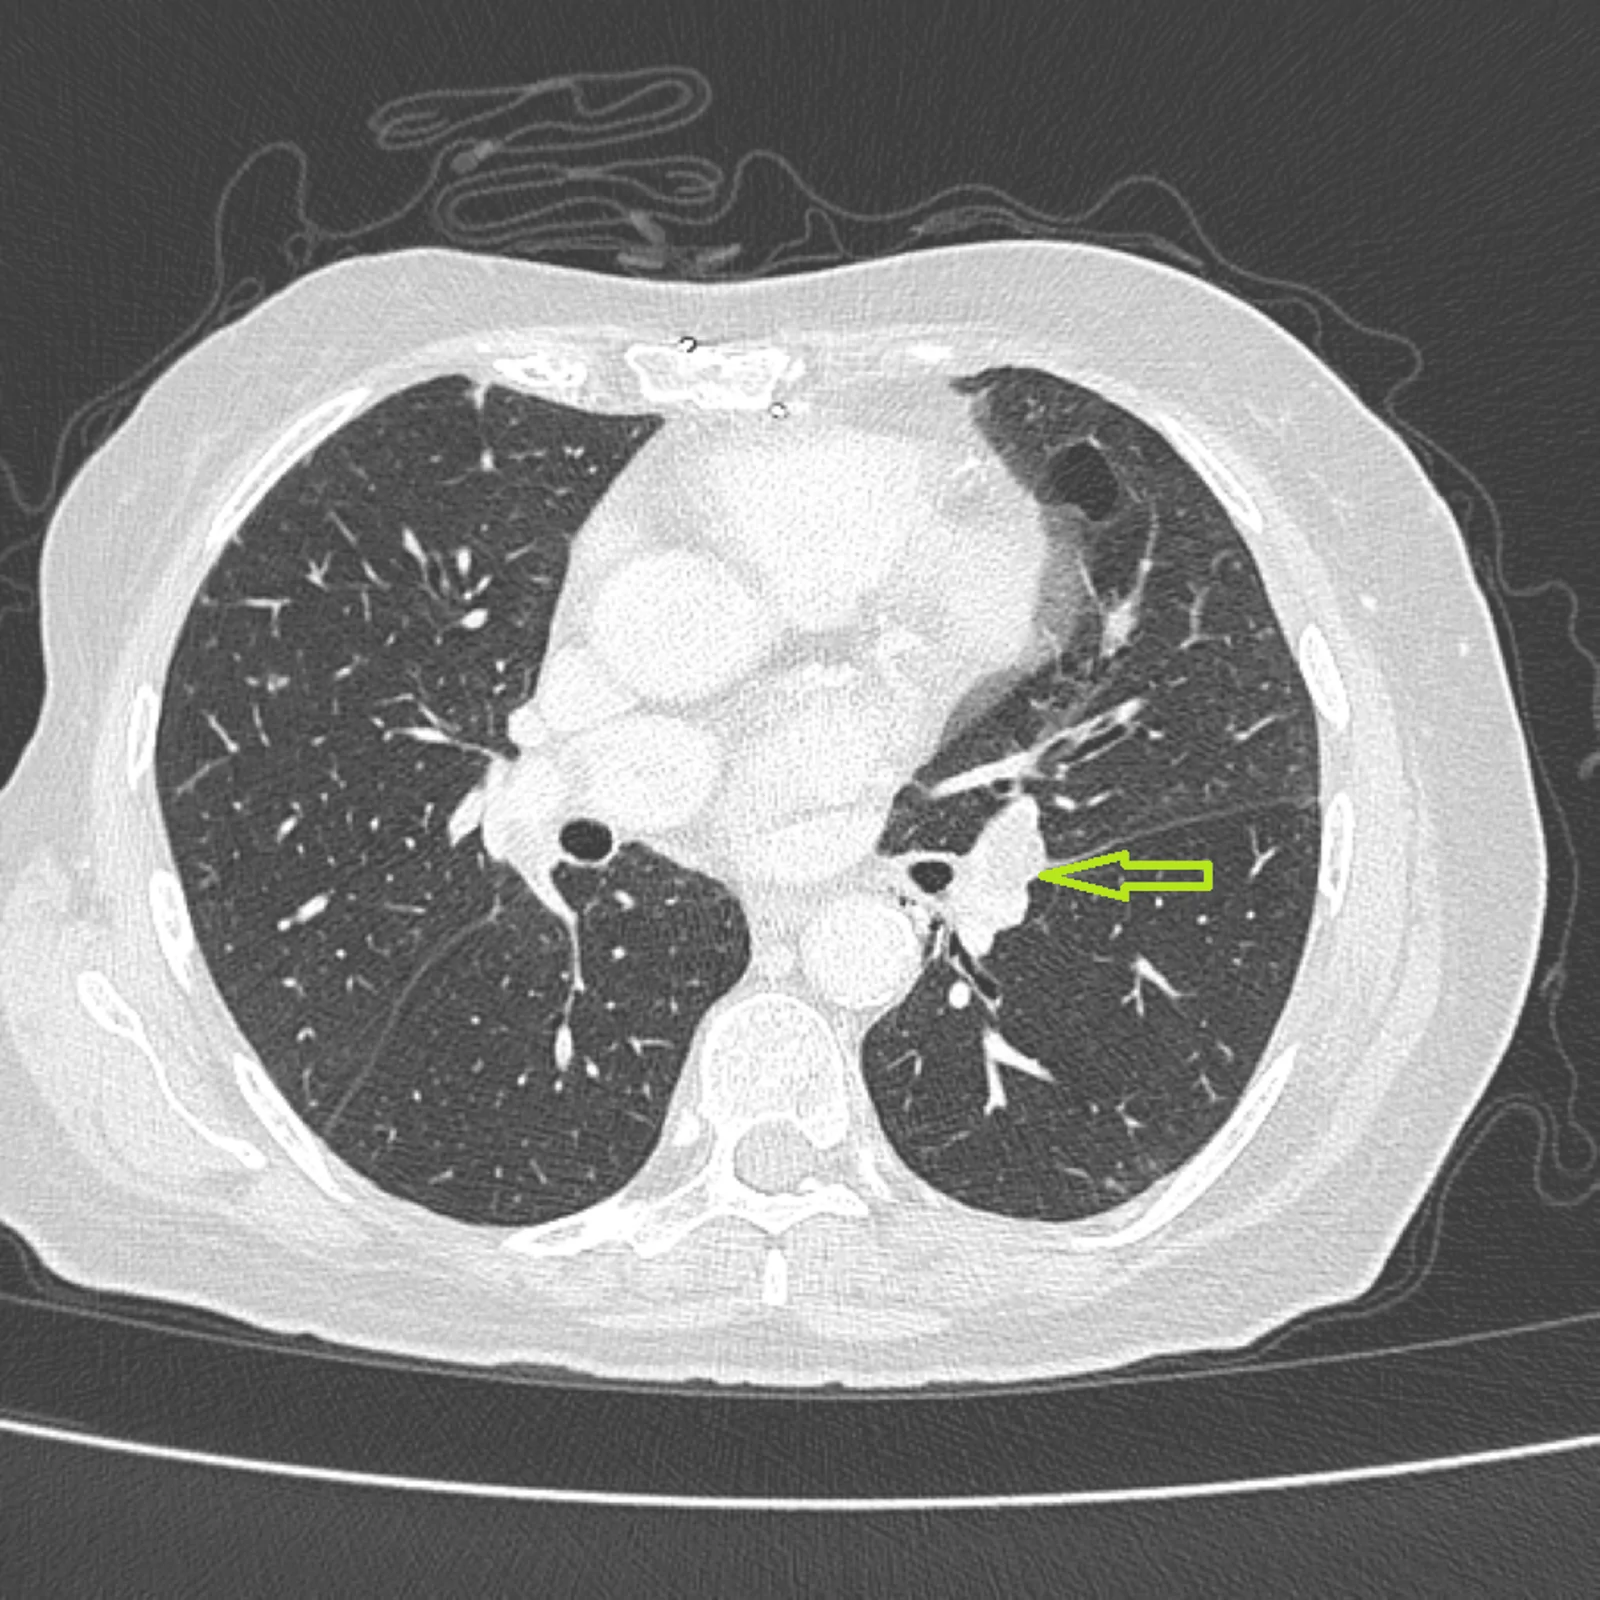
Cross-sectional image of CT thorax with contrast, the green arrow pointing towards the largest visible lymph node (reactive vs. metastatic deposit).
